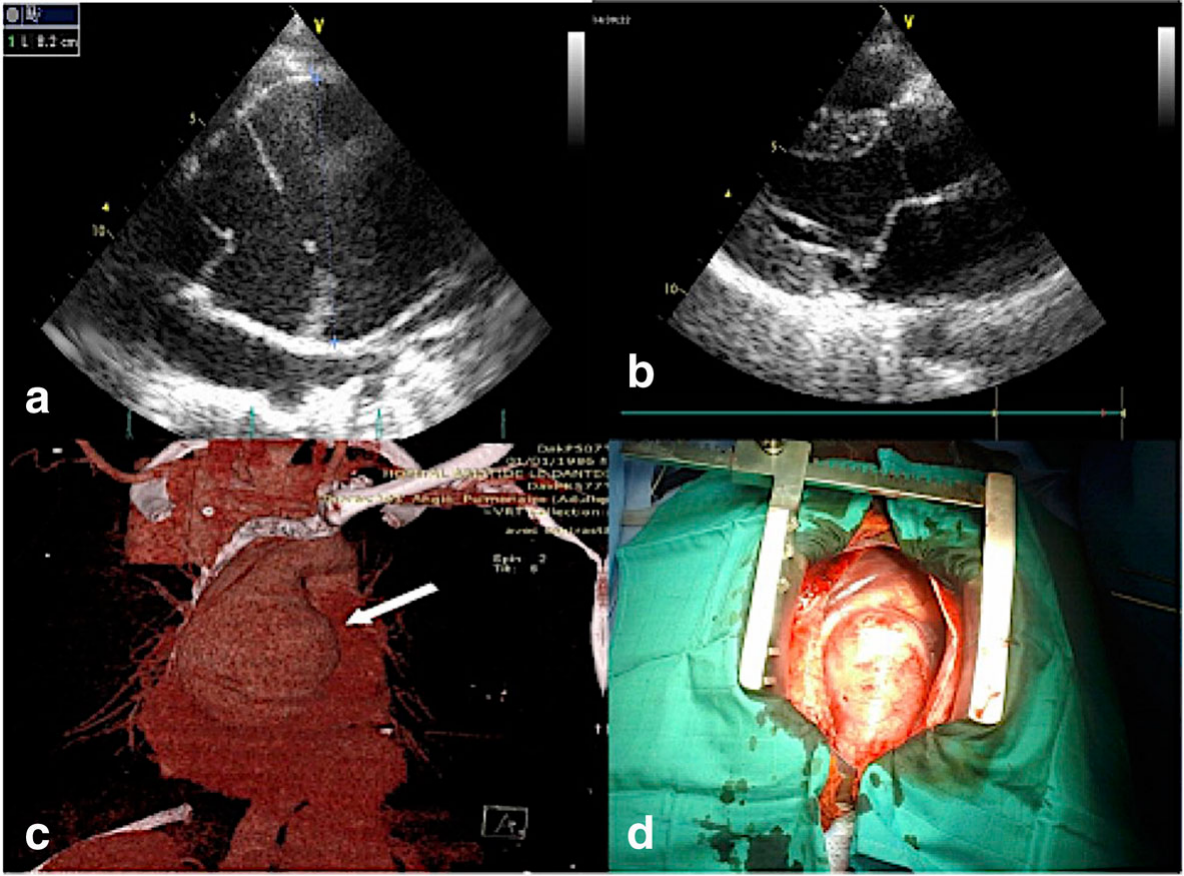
Images of aortic dissection of a patient. a Transthoracic echocardiography parasternal long axis view showing the dilation of aorta and the intimal flap; b Transthoracic echocardography parasternal long axis view showing a mitral valve prolapse; c Computed-tomography scan showing an aortic dissection; d Cardiac surgery of the patient
